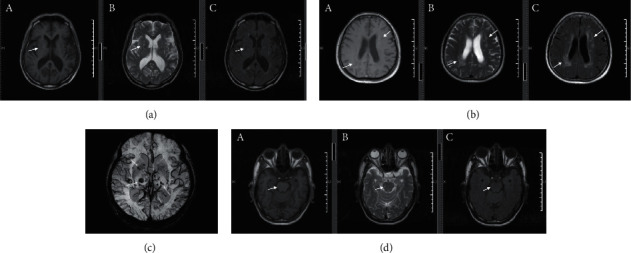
Different image types of CSVD. (a) The lesion showed low signal intensity on T1WI (A); the lesion showed high signal intensity on T2WI (B); the lesion showed low signal intensity on FLAIR (C). (b) Low signal in the anterior and posterior horns of the lateral ventricle on T1WI (A); high signal in the anterior and posterior horns of the lateral ventricle on T2WI (B); high signal in the anterior and posterior horns of the lateral ventricle on FLAIR (C). (c) Lesions showed a low signal on SWI. (d) The brainstem showed a low signal on T1WI (A); the brainstem showed a high signal on T2WI (B); the brainstem showed a high signal on FLAIR (C).
